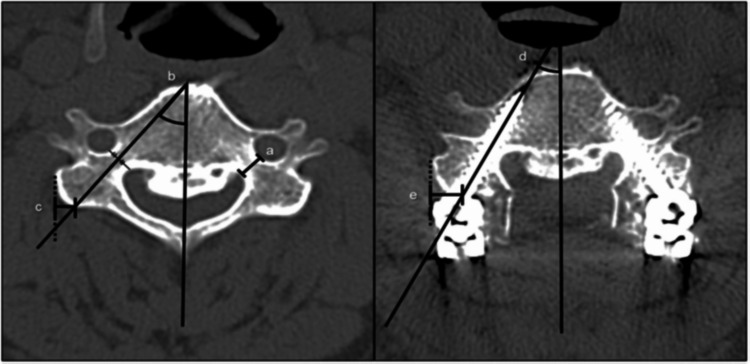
Radiographic parameters of vertebrae and pedicle screw: (a) pedicle width, (b) pedicle transverse angle, (c) pedicle medial offset, (d) pedicle screw transverse angle, (e) pedicle screw medial offset
